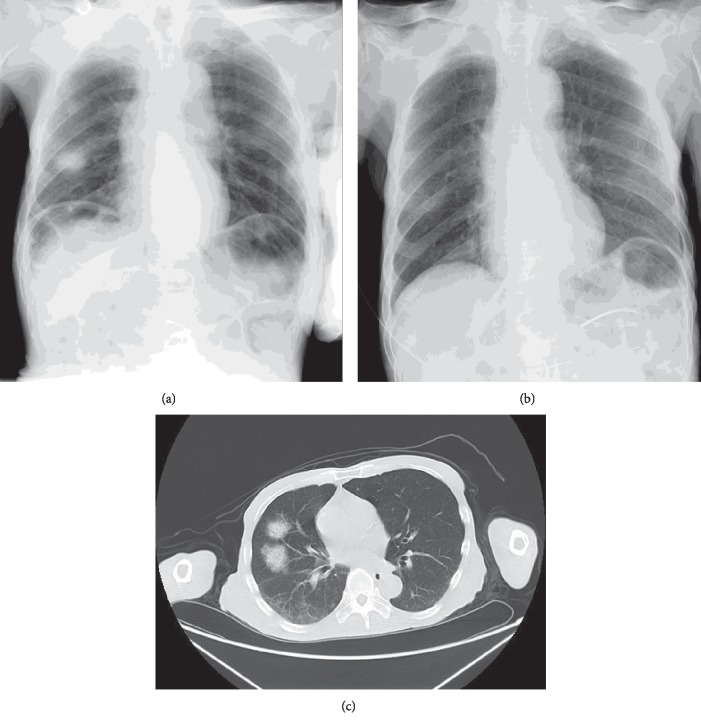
Plain X-ray and CT image. Reticular shadow with ground-glass opacity was observed in the medial lobe of the right lung in the plain X-ray (a) and CT (c) on abnormalities disappeared 45 days later (b).
